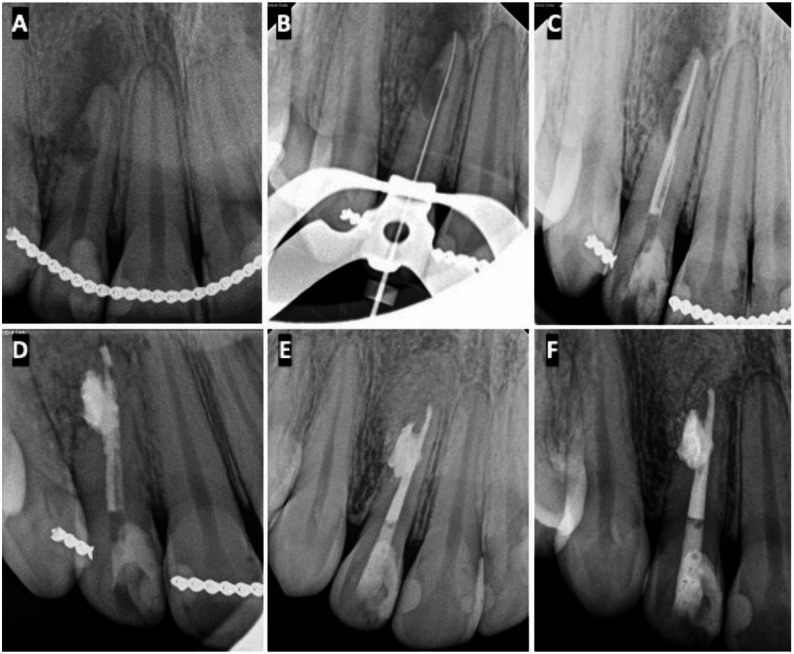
Radiographic sequence demonstrating the diagnosis, treatment procedures, and long-term outcome of perforating internal root resorption in a maxillary lateral incisor (tooth #7). A Preoperative periapical radiograph showing a well-defined radiolucent enlargement of the root canal space in the middle third of the root, consistent with internal root resorption and suggestive of possible perforation of the root wall. A periapical radiolucency associated with the same tooth is also evident. B Working length determination during endodontic treatment using an intracanal file, confirming canal negotiation and preparation. C Placement of calcium hydroxide as an intracanal medicament. D Post-surgical periapical radiograph demonstrating repair of the perforating resorptive defect using a calcium silicate-based bioceramic material following surgical access, debridement, and bone graft placement. Root-end cavity preparation and retrograde filling were also performed under magnification during the surgical procedure. E Immediate postoperative radiograph after completion of root canal obturation and coronal restoration, confirming adequate sealing of the canal system and repair site. F Eight-year follow-up radiograph demonstrating complete resolution of the periapical radiolucency and stable repair of the resorptive defect, indicating long-term clinical and radiographic stability
